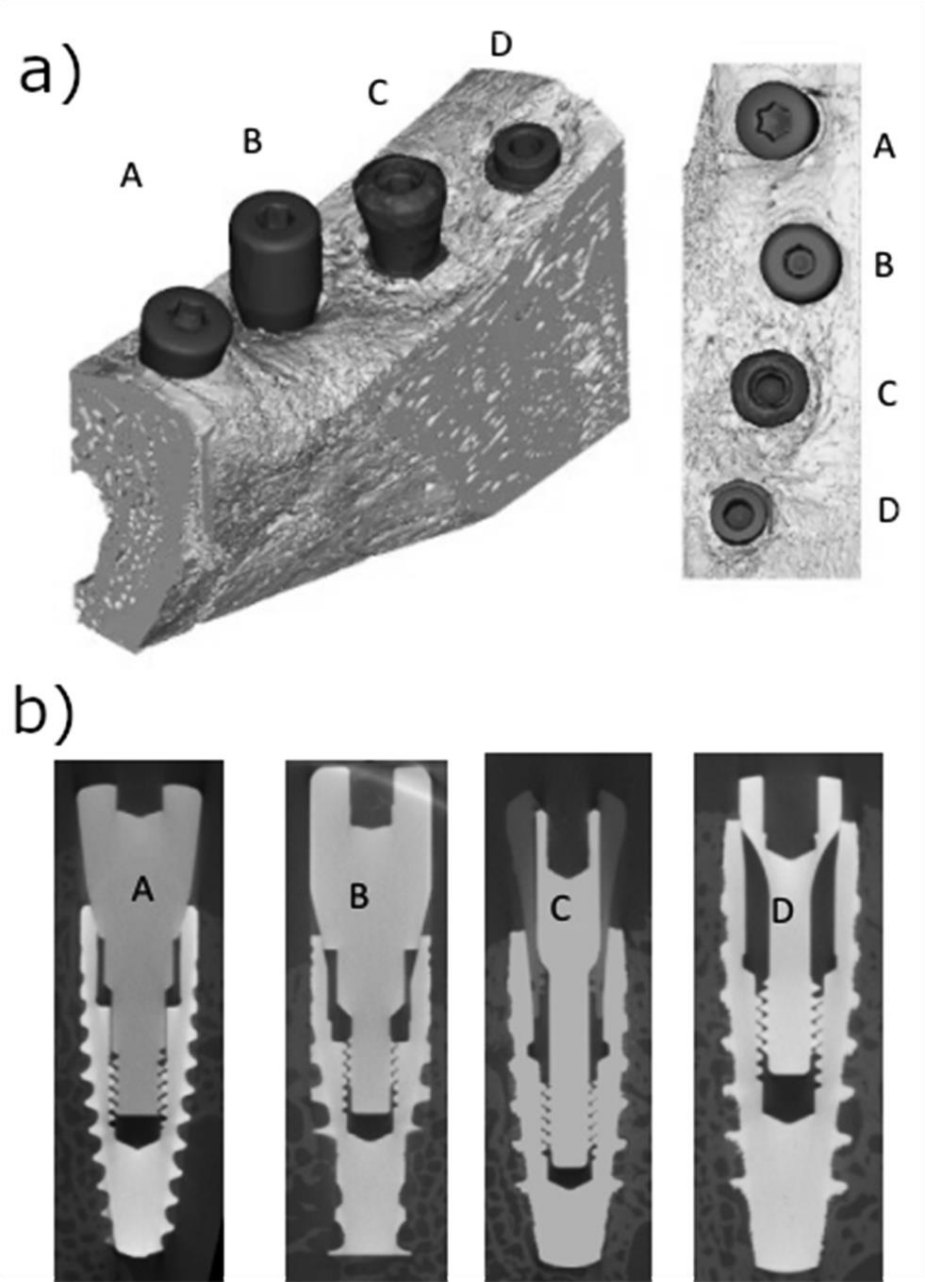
(A) Three-dimensional reconstruction (oblique and top view) of micro CT scans obtained at the test side, with induced periimplantitis, showing a similarity in the marginal bone loss pattern for the tested implant and commercially available brands. (B) Micro CT sample images of implants placed in minipigs' mandibles, with periimplantitis (test). Implants A, B, C, and D are Straumann, Nobel, Plenum, and Plenum submerged (negative control).
